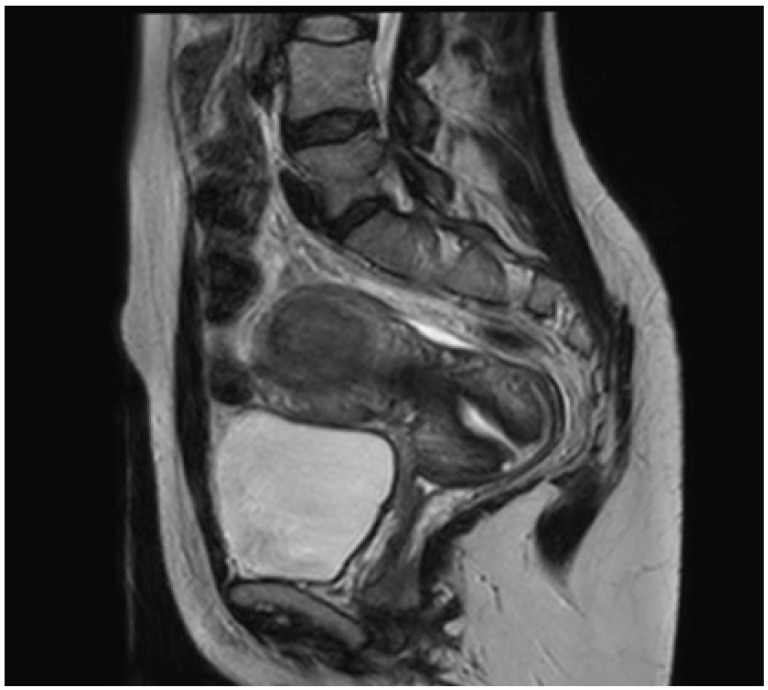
Magnetic resonance imaging of the UTROSCT in Case 1.
